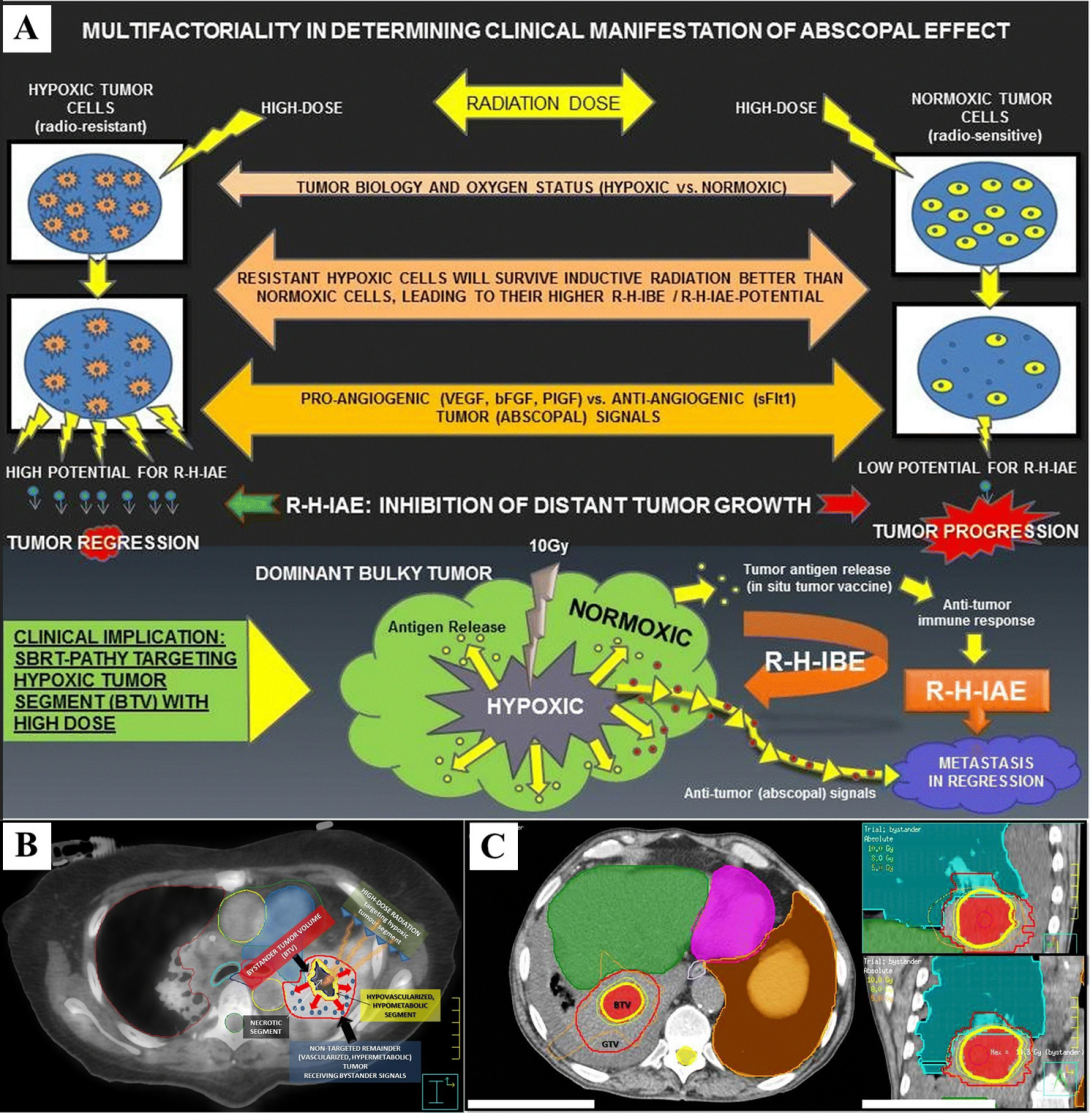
Radiation-hypoxia-induced bystander effect (BE) and abscopal effect (AE). (A) The hypoxic tumor cells shows higher “abscopal potential” than did the normoxic tumor cells, probably due to multiple factors: their higher survival following inductive radiation (10 Gy), radiation dose, tumor biology, oxygen status, and balance between pro-angiogenic and anti-angiogenic “abscopal messengers”; (B) definition of the bystander tumor volume (BTV): an 18F-FDG PET combined with a contrast-enhanced CT was used for the definition of BTV (smaller yellow contour), which corresponds to the junctional region between the central necrotic segment (black region) and the contrast-enhanced, hypermetabolic peripheral tumor (red contour, not targeted for irradiation). The red arrows represent “anti-angiogenic bystander signal” (blue pellets) released by the irradiated hypoxic tumor, inducing the regression of the non-targeted tumor; (C) SBRT-PATHY dose distribution: a large lung bulky tumor (GTV, red contour) irradiated partially by targeting exclusively the BTV (bystander tumor volume-hypoxic segment) with 10 Gy (yellow isodose-line) in single fraction to the 70%-isodose line (Dmax 14.3 Gy). Green and orange isodose-lines correspond to 8 Gy and 5 Gy, respectively. SBRT: stereotactic body radiotherapy *Note.*
